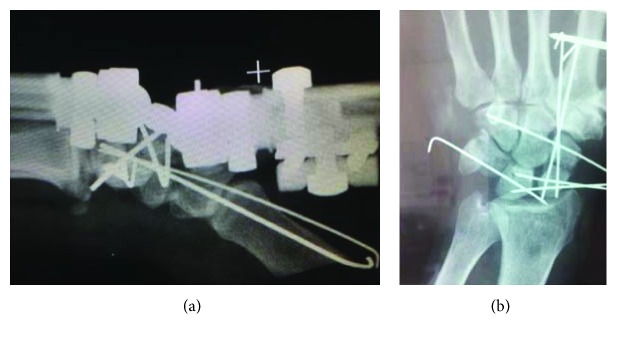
Immediate postoperative X-rays of the left wrist showing open reduction and internal fixation with Kirschner wires and external fixation.
